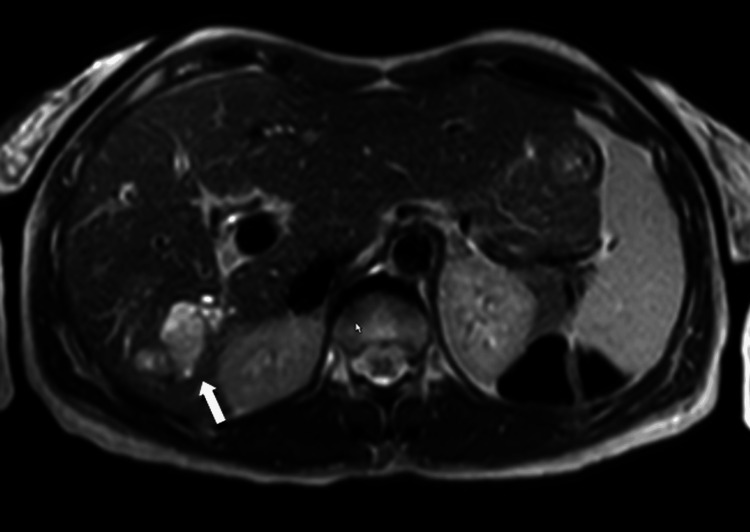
Axial T2-weighted MRI image at the level of the lesion Axial T2-weighted MRI demonstrating a papillary intraductal mass within a dilated bile duct in segment VI, appearing slightly hyperintense compared with the surrounding liver parenchyma (white arrow).
